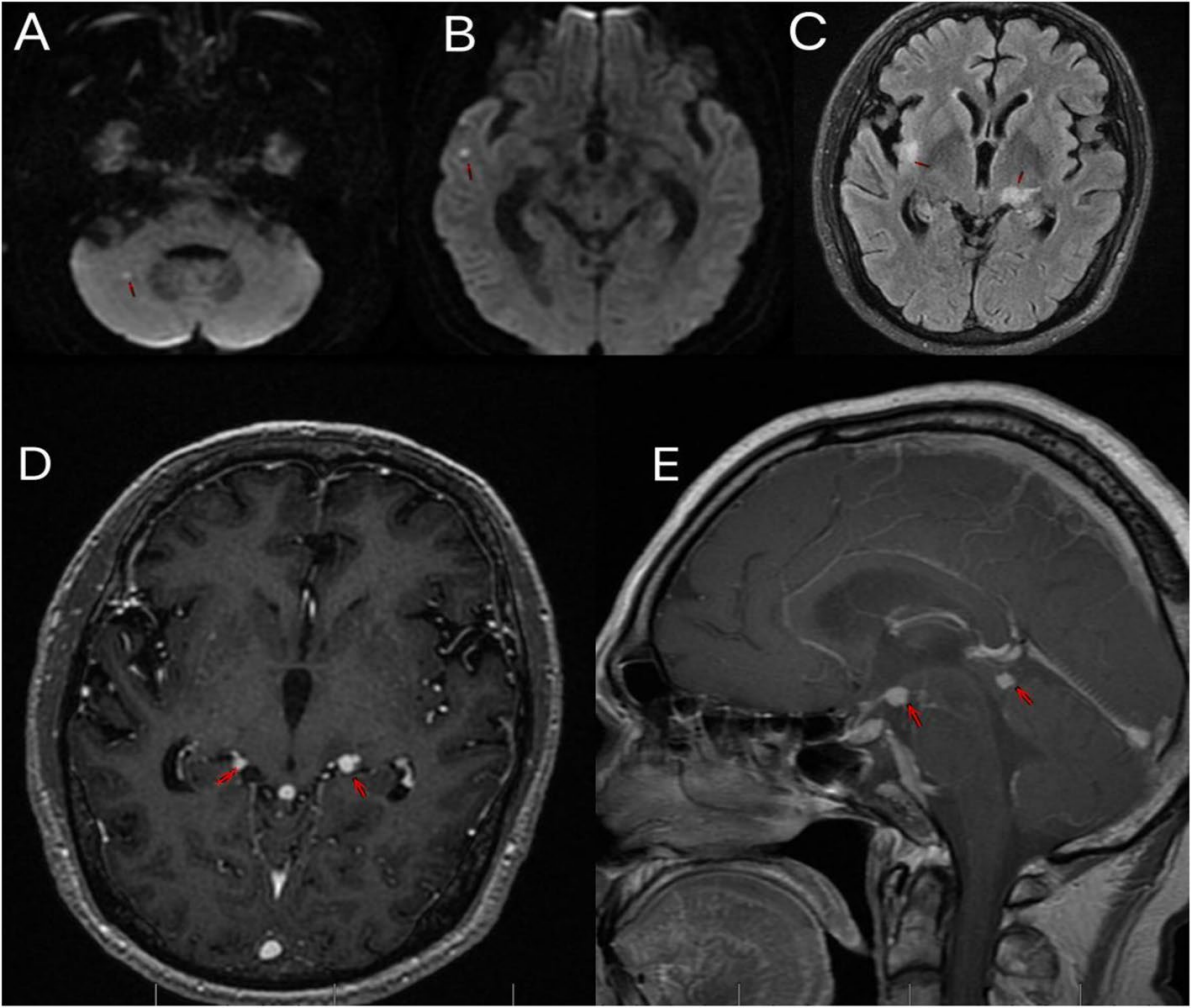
A-B: Axial Diffusion-Weighted Imaging (DWI) revealing punctate hyperintense lesions in the right cerebellar hemispheres and the temporal lobe. C: Axial T2-Weighted-Fluid-Attenuated-Inversion-Recovery (FLAIR) imaging revealing hyperintense lesions in the right insula and the left thalamus. D: Axial T1-Weighted contrast-enhancement imaging revealing significant circle enhancement lesions in the ambient cistern and quadrigeminal cistern. E: Sagittal T1-Weighted contrast-enhancement imaging revealing significant enhancement lesions in the ambient cistern and prepontine cistern
